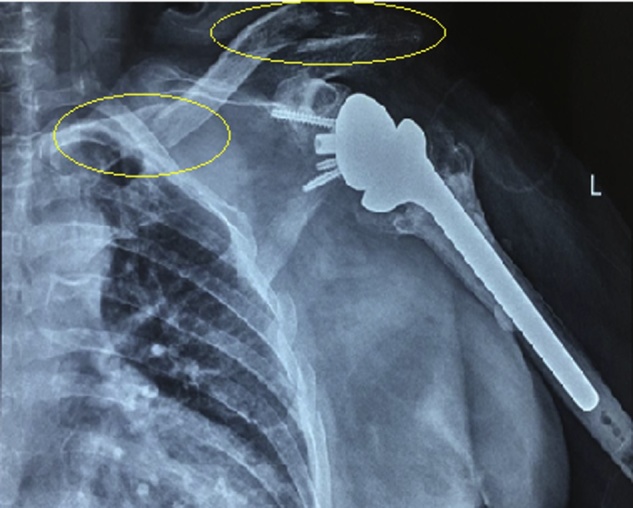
X-ray radiograph of the left clavicle and shoulder joint showing a proximal and distal clavicle fractures with intact reverse shoulder prosthesis in good alignment.
